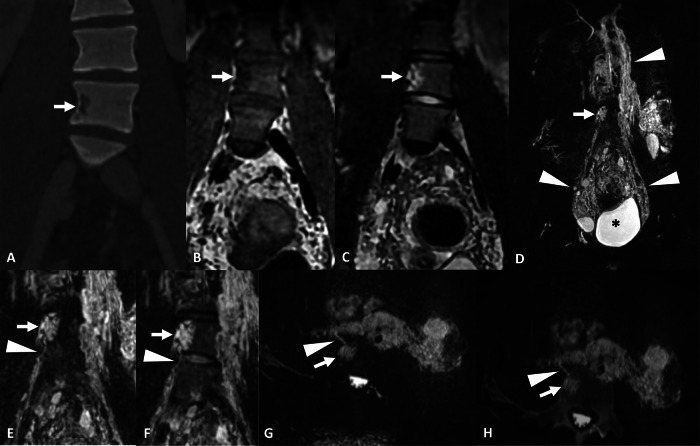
Initial work-up of a buttock and thigh swelling in a 16-year-old male revealing GLA with intraosseous noncystic lymphangioma. A Noncontrast CT, coronal view. Juxtacortical lesion of vertebra with low attenuation density and without cortical destruction (arrow). B, C T1w and STIR, respectively, coronal view. Juxtacortical lobulated vertebral lesion, with irregular borders, low signal intensity on T1w images and high signal intensity on STIR images. Note the few high signal intensity spots on T1w images disappearing on STIR images corresponding to intralesional fat (arrow). D 3D FRFSE T2w, MIP reconstruction (20 mm), coronal view, revealing a bulky pelvic lymphangioma (*) associated with retroperitoneal and pelvic lymphangiectasis (arrowheads). Note the vertebral noncystic lymphangioma (arrow). E, F 3D FRFSE T2w, MIP reconstruction (20 mm), coronal view and fusion between NEMRL and STIR image. Identifies a connection (arrowhead) between the vertebral noncystic lymphangioma (arrow) and the paravertebral retroperitoneal lymphangiectasis. In the presence of a primary lymphatic disease, characterized by lymphatic proliferation, the connections between the intraosseous and extraosseous lymphatics become hyperplastic and dysplastic and hence visible on NEMRL. G, H 3D FRFSE T2w, MIP reconstruction (20 mm), axial view and fusion between NEMRL and noncontrast CT image. The connection (arrowhead) between the vertebral noncystic lymphangioma (arrow) and the paravertebral retroperitoneal lymphangiectasis is also well visualized on axial images. Note the paravertebral retroperitoneal lymphangiectasis sheathing the inferior vena cava and the abdominal aorta
